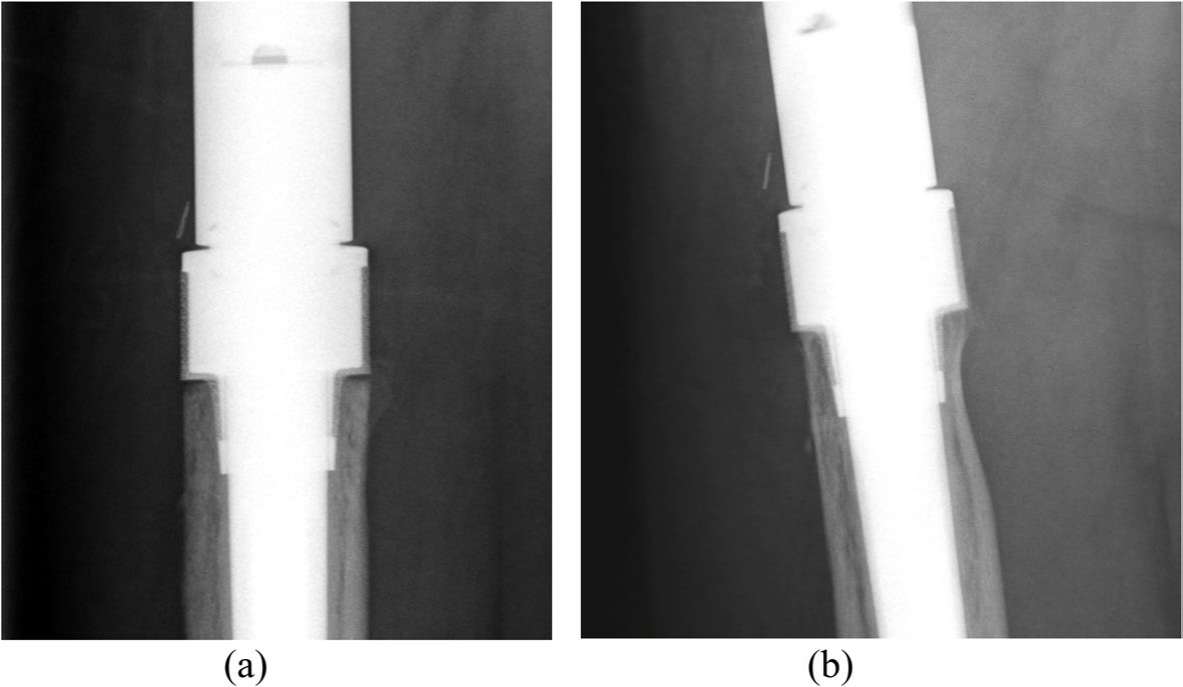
Example of radiographic ongrowth score for endoprostheses (ROSE) using the porous bridging collar: a radiograph immediately post-implantation showing radiolucencies between the osteotomized bone and the extra-osteal portion of the porous collar; b absence of radiolucencies between the host bone and the collar medially and laterally, indicating a ROSE score of + 2 on this radiograph
